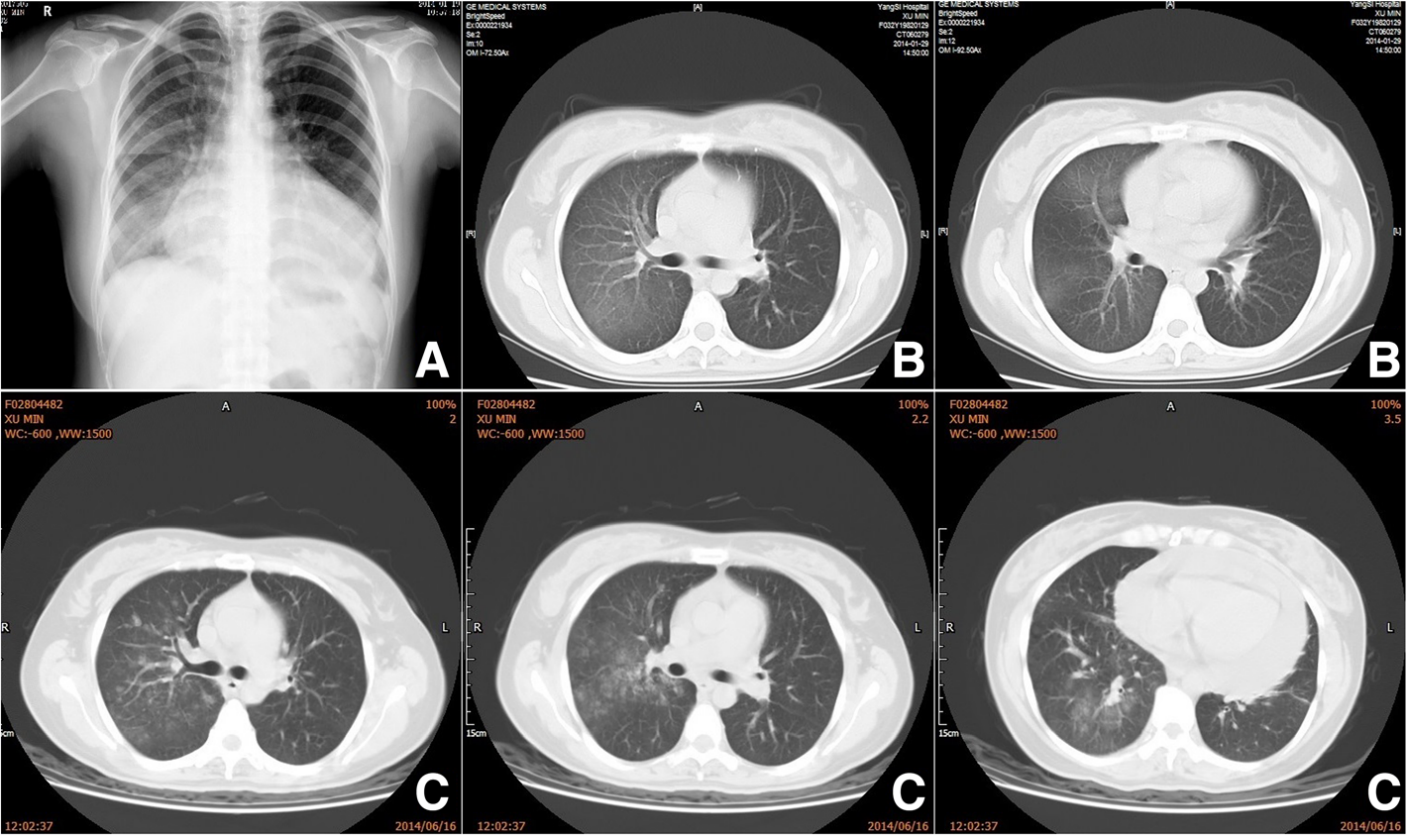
Chest x-ray and CT image of the chest obtained at admission showing ground-glass opacities in the right lobes. a Chest x-ray obtained on January 19, 2014; (b) CT images of the chest obtained on January 29, 2014; (c) CT image obtained on June 16, 2014
